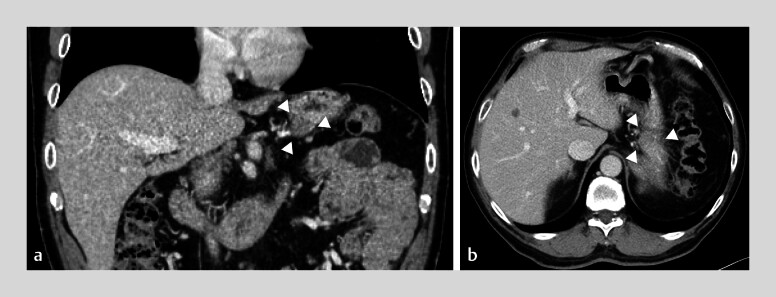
Needle tract seeding (NTS) along the endoscopic ultrasonography-guided fine-needle aspiration (EUS-FNA) puncture route: Imaging findings 2.5 years after initial EUS-FNA for pancreatic body adenocarcinoma (PDAC).
